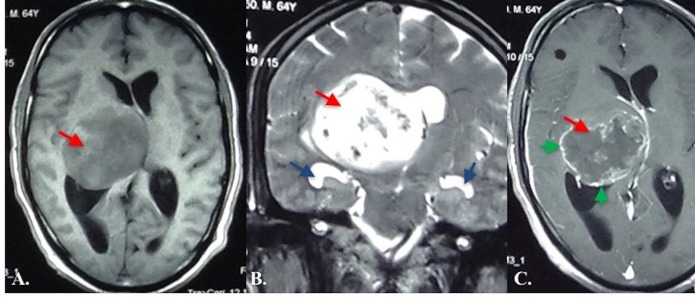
Preoperative Magnetic Resonance Imaging of the Patient T1-weighted (A), T2-weighted (B), and T1 after Gadolinium (C) show a high-grade lesion in right thalamus (red arrow) with peripheral contrast enhancement (green arrow). Note that the patient is getting hydrocephalic as temporal ventricular horns are enlarged (blue arrows).
